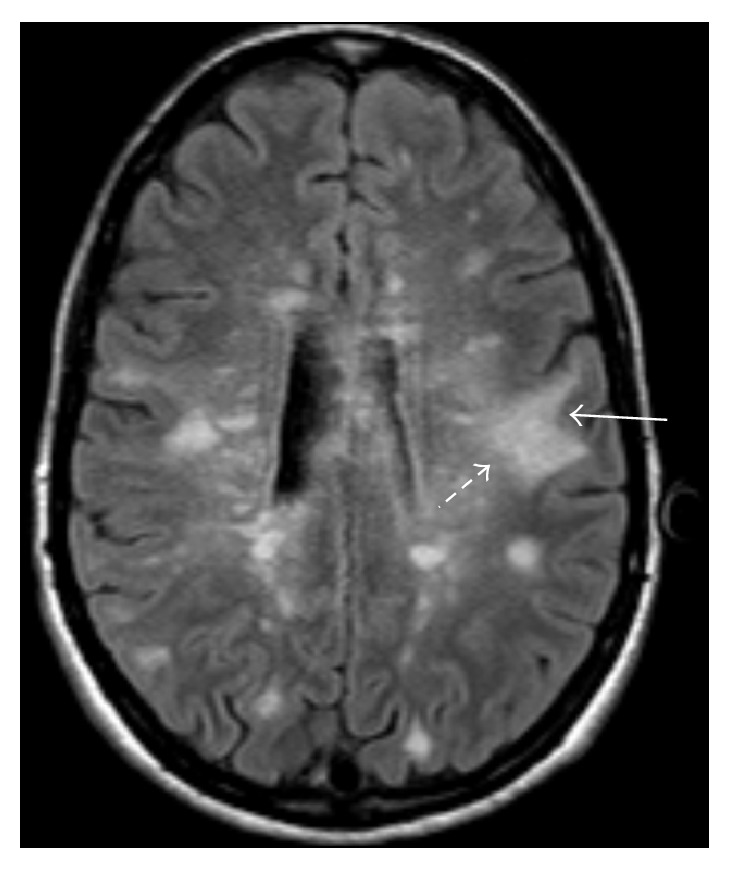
25-year-old woman with RRMS who developed worsening symptoms including weakness and inability to ambulate after beginning natalizumab. MRI FLAIR image demonstrates classic appearance of PML including a sharply demarcated peripheral border along the subcortical U-fibers (arrow) and a hazy, ill-defined central border (dashed arrow).
